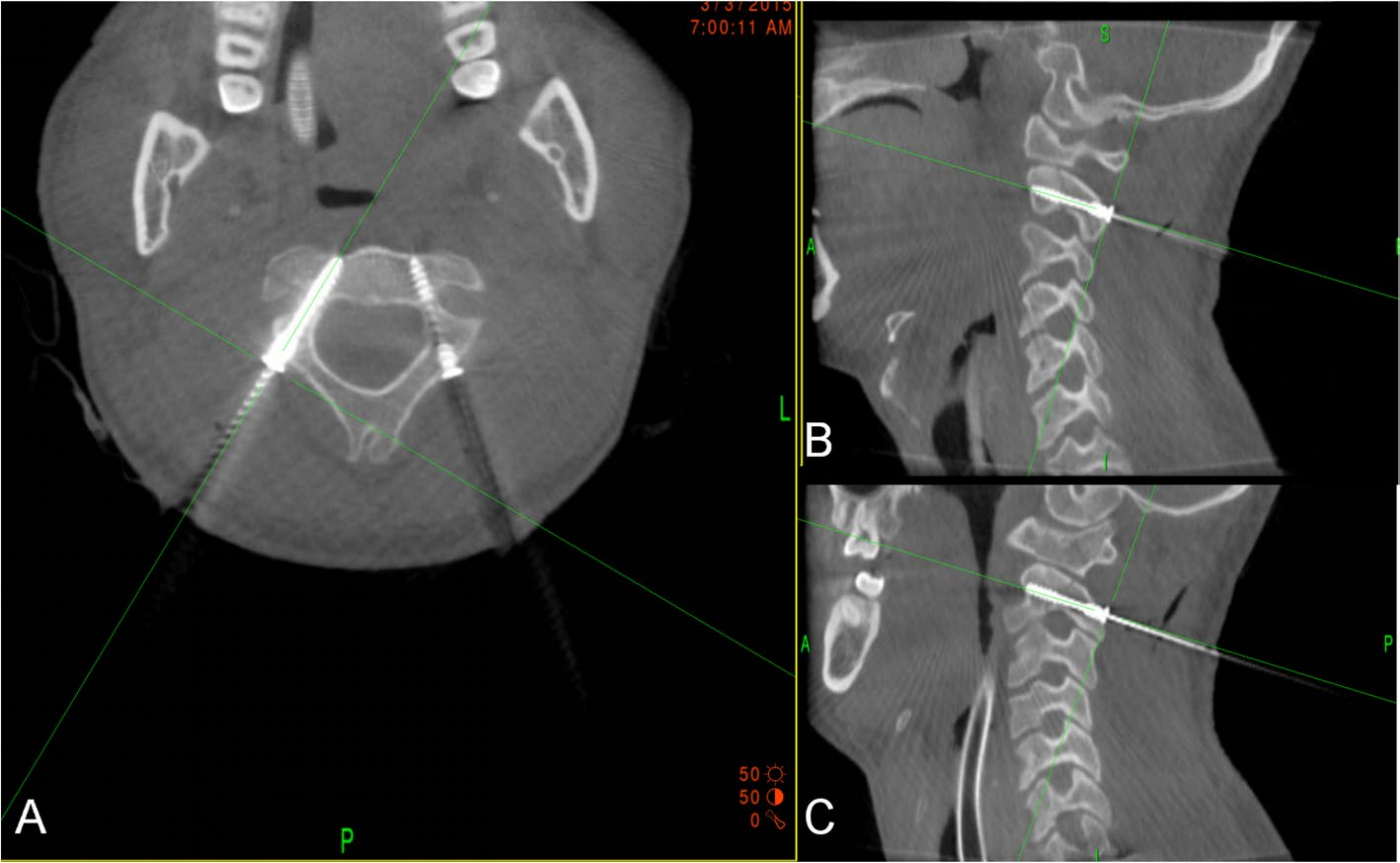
Intraoperative computed tomographic CT scans showing right-sided C2 pedicle screw in axial and sagittal planes (A, C). Intraoperative CT scans showing left-sided C2 pedicle screw in axial and sagittal planes (A, B)
